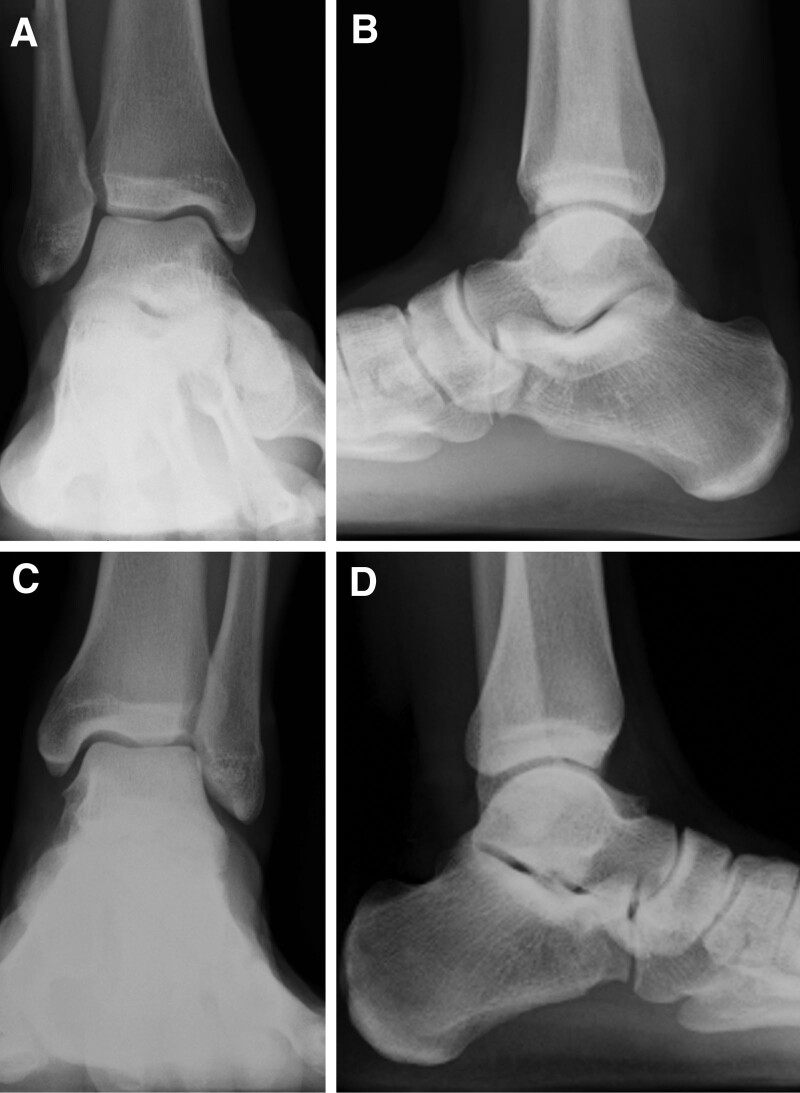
Preoperative radiographs of the right (A, B) and the left (C, D) feet show no obvious abnormal findings.
